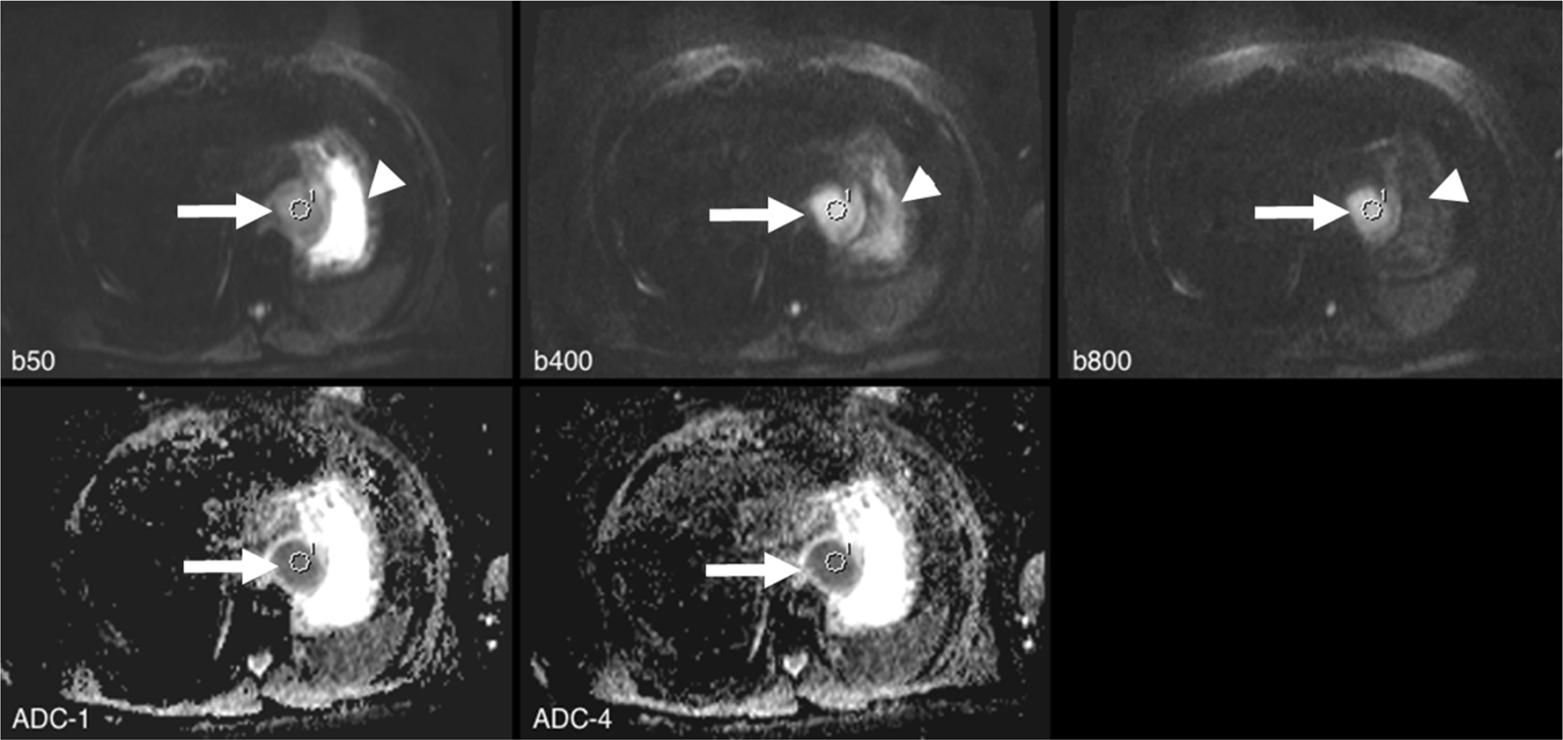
Examples of reading approaches: A 55-year-old male with advanced-stage oesophageal cancer (arrow). The image shows the reading set-up, with raw diffusion-weighted imaging (DWI) data on the top row (b50, b400, b800 [s/ mm^2^]), and, below, parametric apparent diffusion coefficient (ADC) maps of ADC_1 and ADC_4. The latter were automatically generated inline by the scanner or by the viewing workstation (MMWP, details see above). On raw DWI data, the typical signal decay of free water can be depicted in the adjacent stomach, whereas the lesion itself shows signal alteration indicative of diffusion restriction (arrowhead). The corresponding ROI 1 was placed within the tumour in the b800 image and ROI coordinates were automatically transferred to all other remaining series. This approach excluded user-dependent bias when comparing the ROI statistics between the given series
